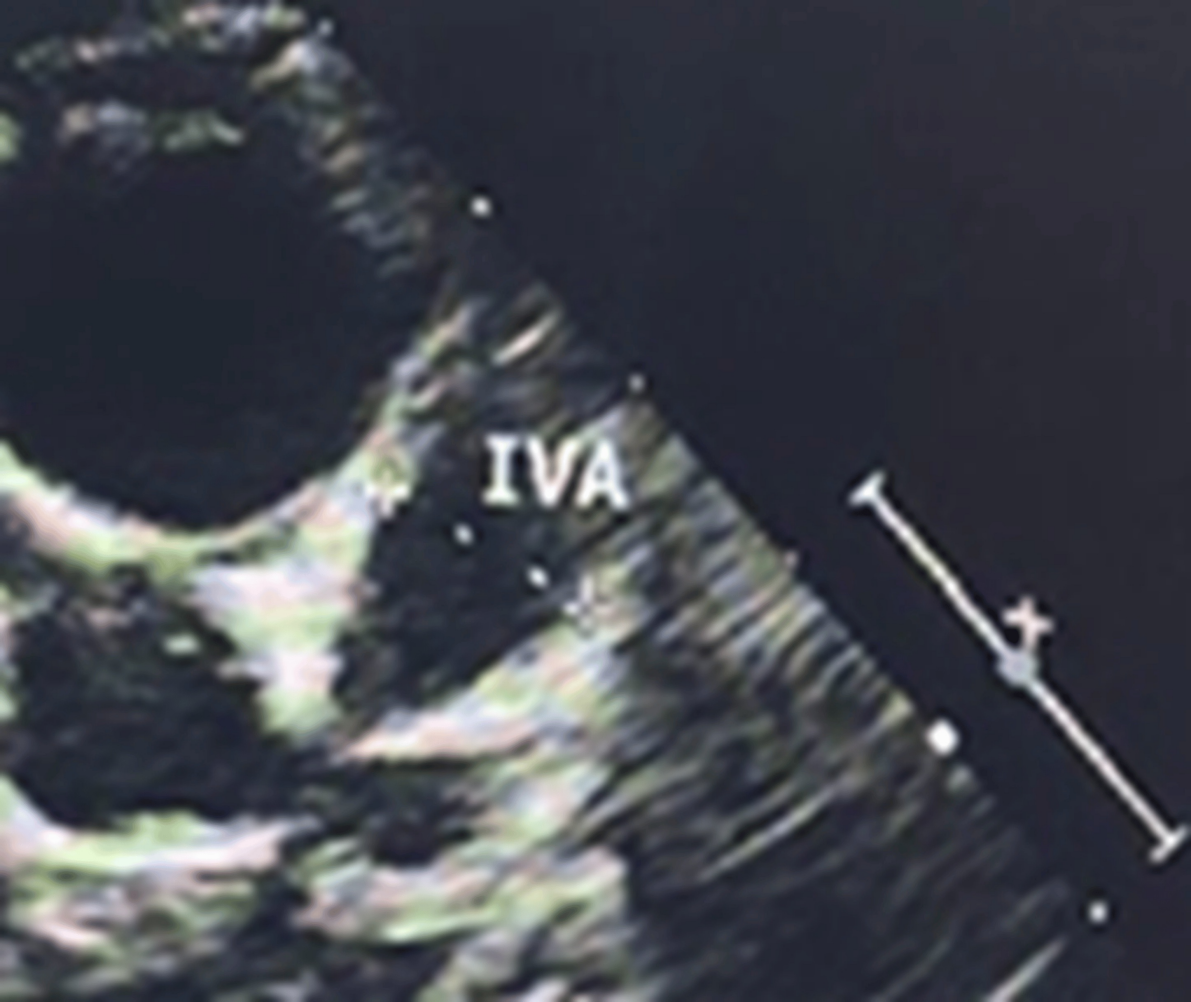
Transthoracic echocardiography revealing aneurysms of the left anterior IVA: isovolumic acceleration
